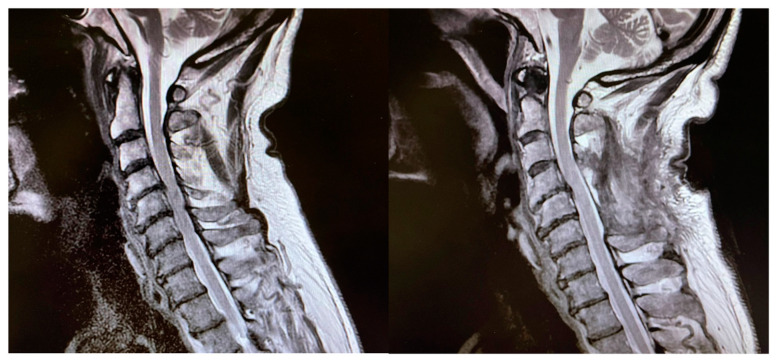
On the left, pre-operative T2 MRI image showing spinal cord compression due to degenerative cervical spondylosis; on the right, post-operative T2 MRI image showing satisfactory decompression of the spinal cord after multi-level laminectomy.
